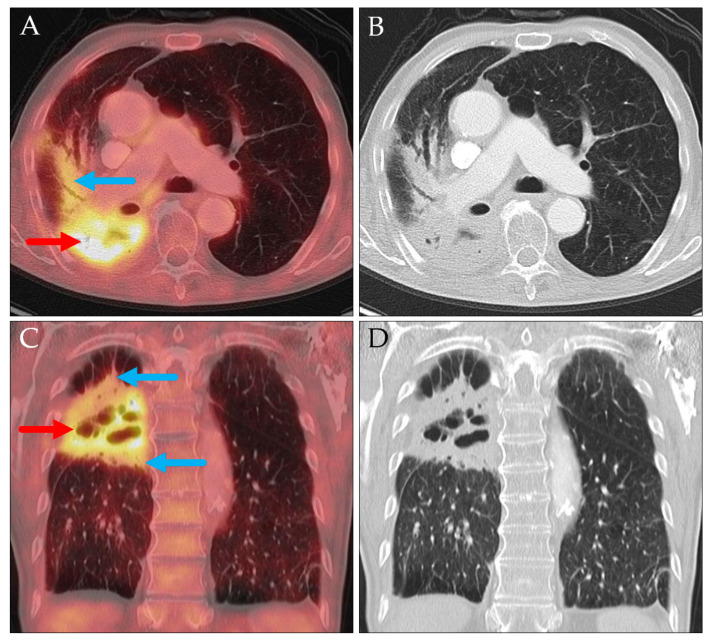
Axial PET/CT (A) and CT (B); coronal PET/CT (C) and CT (D). Primary lung carcinoma (red arrows) with peritumoral infiltrates/atelectases (blue arrows), which are also hypermetabolic and difficult to differentiate from actual tumor tissue.
